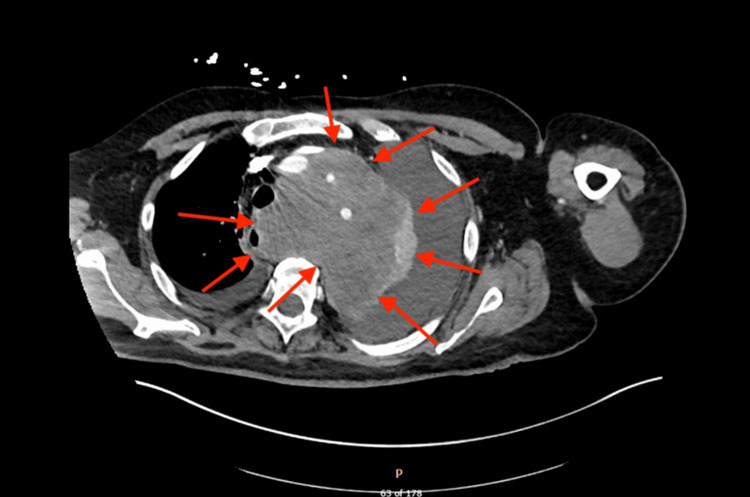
CT scan performed on August 31, 2024, revealing a mediastinal mass that has encased structures in the surrounding tissues
